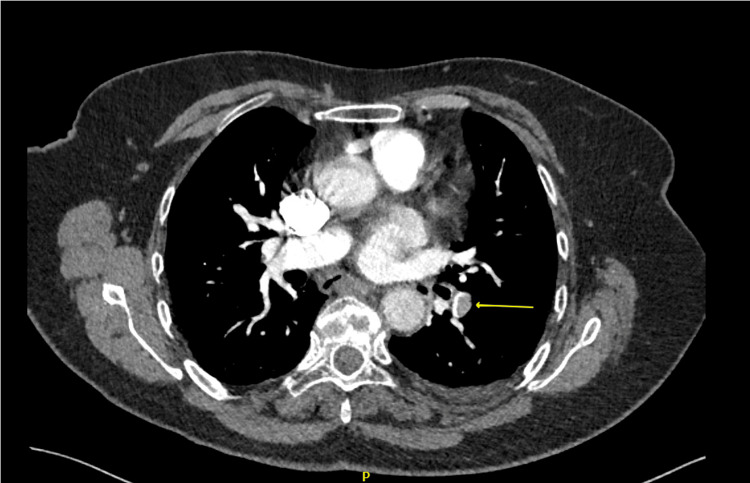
The CTPA showing filling defects in the left pulmonary artery (pointing yellow arrow), suggestive of left-sided PE. CTPA: Computed tomography pulmonary angiography, PE: Pulmonary embolism
